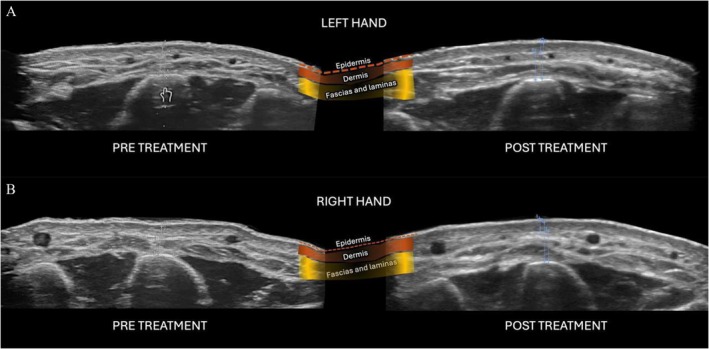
Ultrasound images pre‐ and post‐treatment (Day 90): CaHA + PMN (A) and CaHA + SS (B). Colored overlays highlight skin layers.
